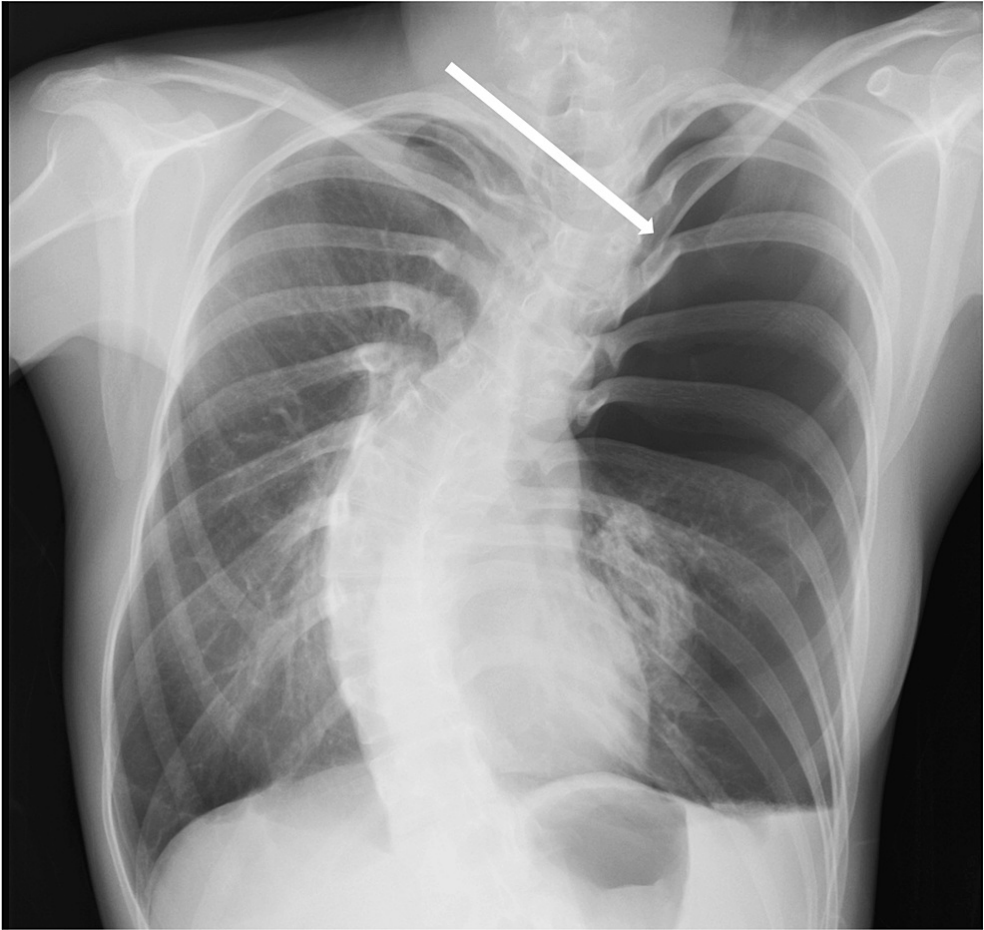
Chest radiograph (frontal view) The chest radiograph shows the collapse of the left lung.
